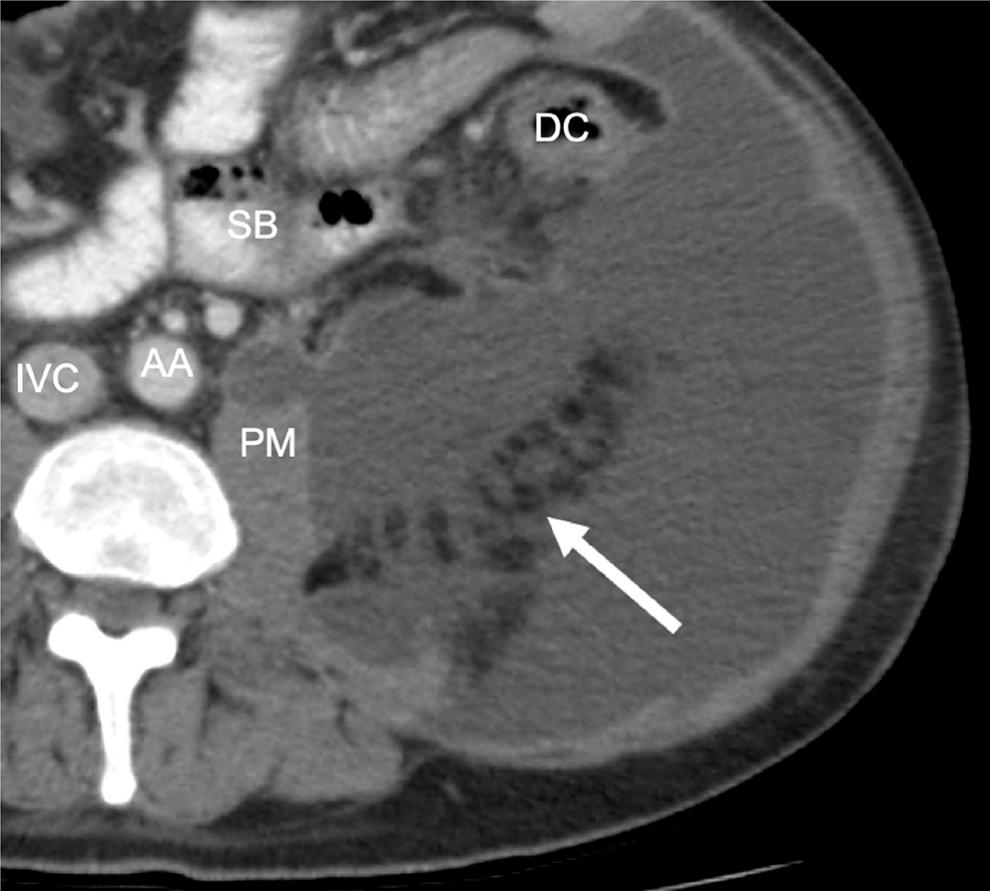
CT image of the left retroperitoneum at the level of the L4 vertebra below the kidneys. A large amount of fluid in the retroperitoneum tracks around and also dorsal to the flank pad (arrow pointing to the flank pad itself). AA: abdominal aorta, DC: descending colon, IVC: inferior vena cava, PM: psoas major muscle, SB: small bowel
